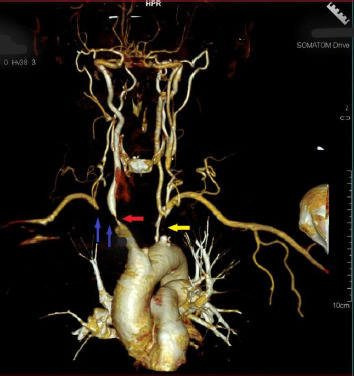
Volume rendered image of CT head and neck angiography. Red arrow: significant narrowing of the right common carotid artery, blue arrow: non-opacification of the proximal right subclavian artery, yellow arrow: non-opacification of the proximal left subclavian artery
